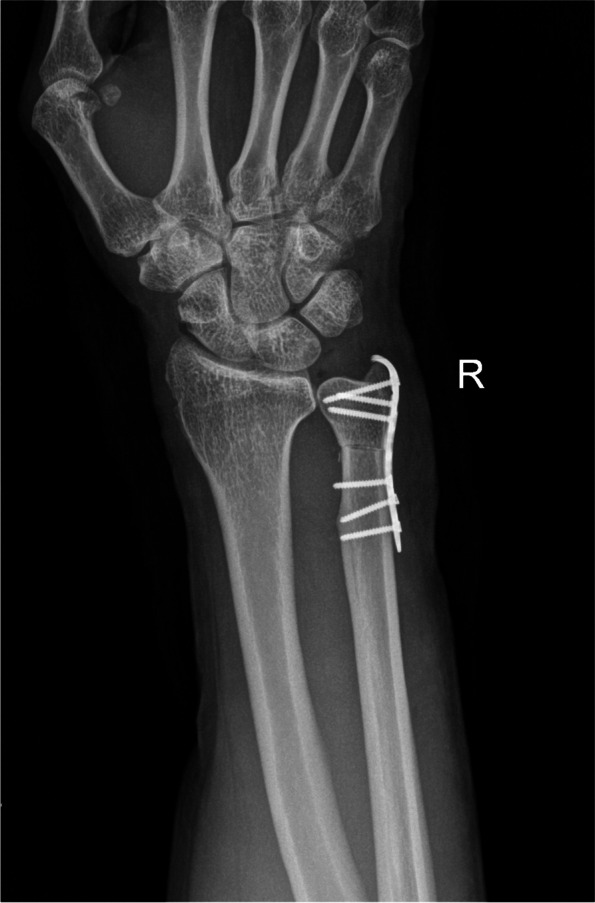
Postoperative radiograph of metaphyseal USO
